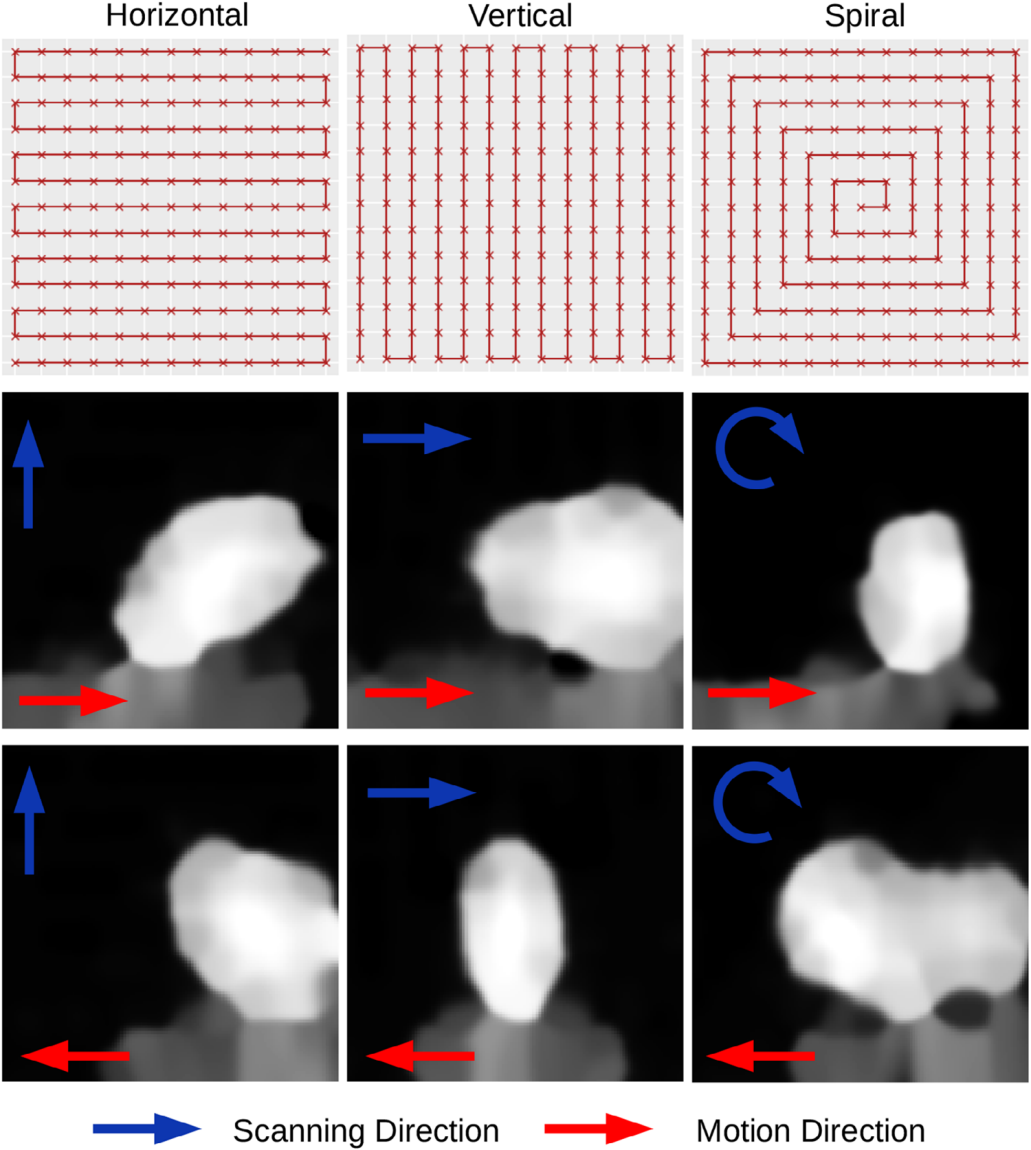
Demonstration of the effect of different scanning patterns on the resulting image when imaging a moving sphere with proton radiography. The first row shows the scanning pattern, The beam started at one end of the red line and scanned following the red line with the ‘x’ set spot positions for a frame. The direction was then reversed for the next frame. The first column shows the effect of imaging in parallel with the direction of motion, the middle shows the effect of sampling perpendicular to the object motion and the third column shows the effect of imaging in a spiral pattern. The direction that the beam moved through the pattern and the direction of motion are indicated by the arrows.
